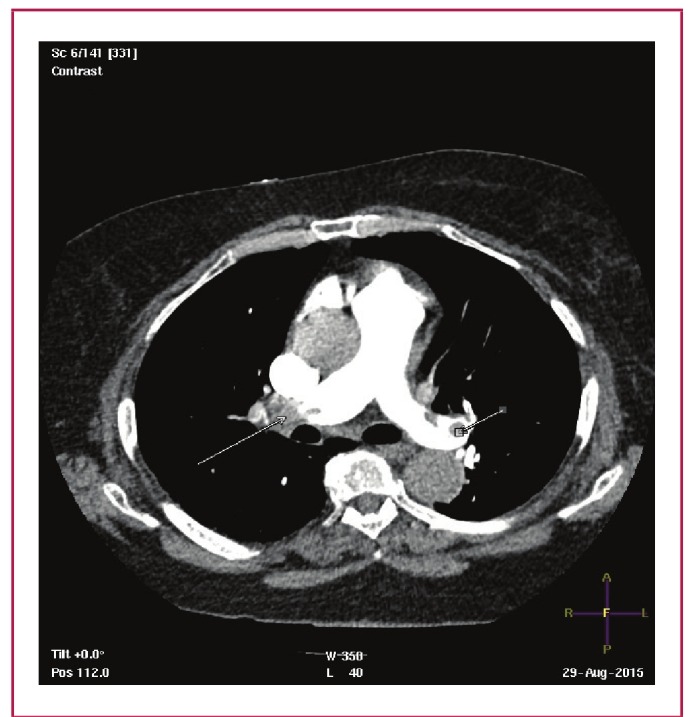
Example of calculation of the Qanadli obstruction index from CTPA. Axial CT scan shows a proximal completely occlusive thrombus (long arrow) in the right main pulmonary artery, and partial thrombus in the left main pulmonary artery (short arrow). The Qanadli score index would therefore be [(10 x 2) + (10 x 1) = 30/40] = 75%.
